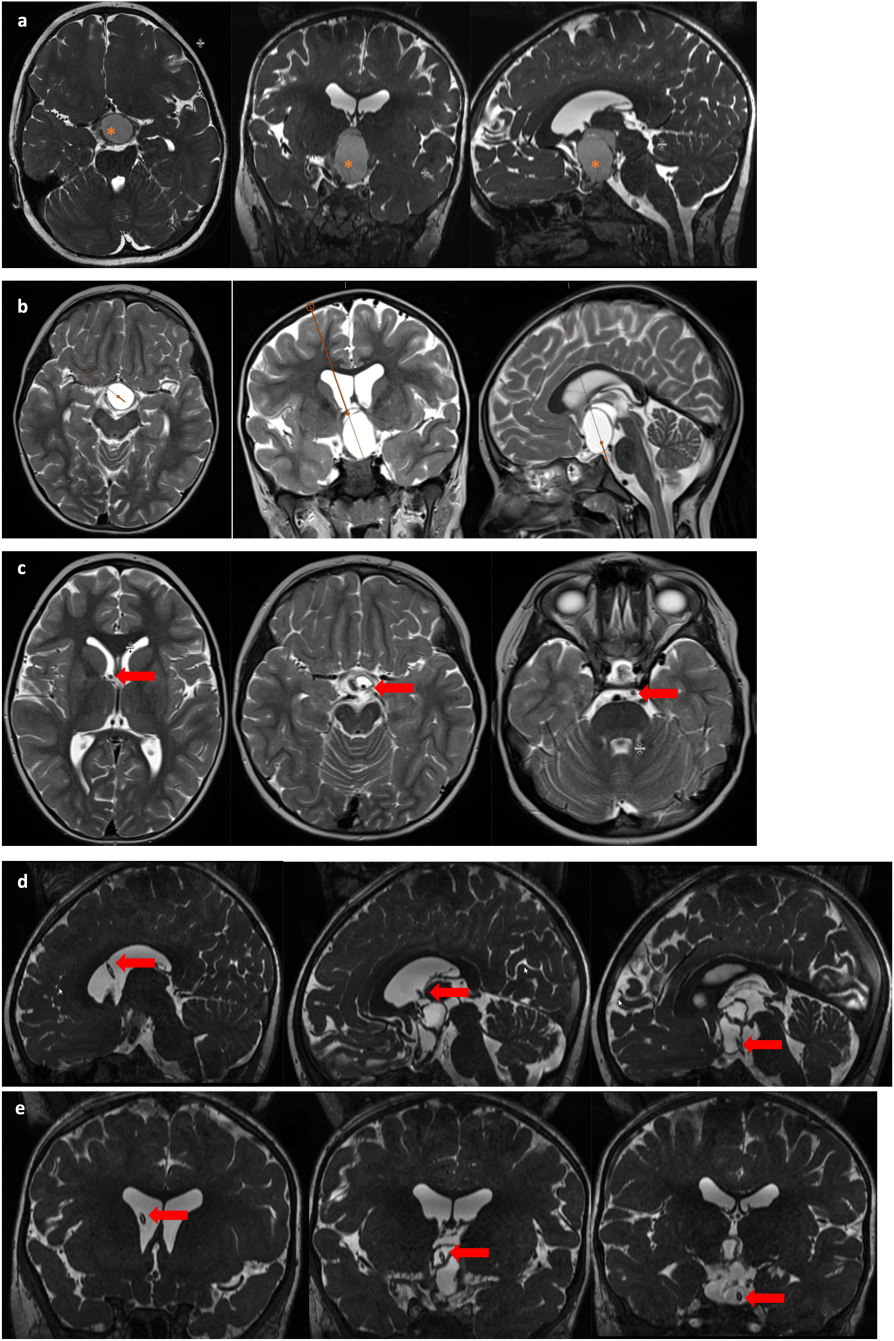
MR imaging of a 5-year old female patient with a suprasellar cystic, space-occupying craniopharyngioma treated with stereotactic implantation of an internal shunt system. This patient presented with initial symptoms of mild visual deficiency and headache. Image line (A) demonstrates preoperative findings with a large intra-/suprasellar cystic tumor (*) compressing the chiasma. After planning the stereotactic trajectory (image line B) for implantation of an internal shunt system, the cystic tumor formation was reduced (image lines C (axial), D (sagittal) and coronal E) resulting in a decompressed chiasm and consecutive improvement of visual function. The arrow marks the course of the internal shunt through the foramen Monroi, the tumor cyst with final position in the prepontine cistern.
